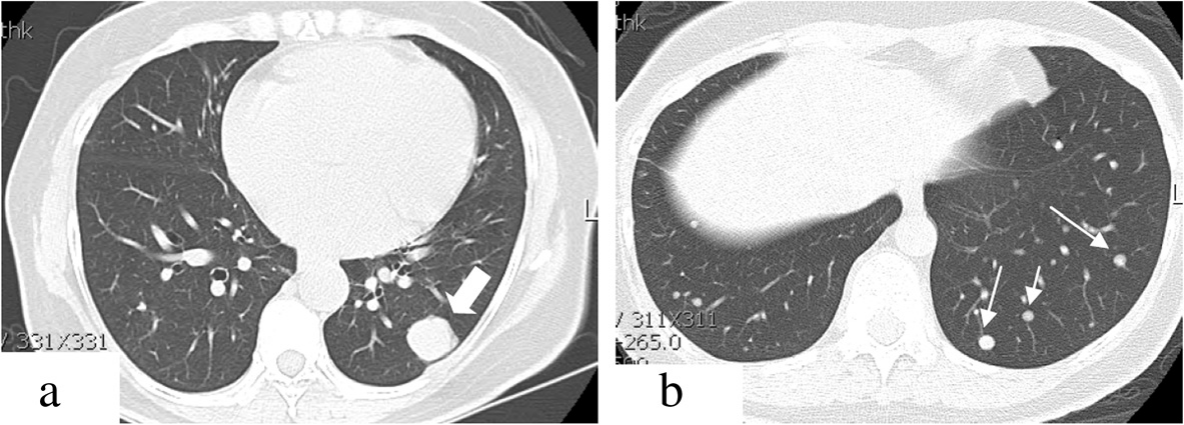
Chest computed tomography (CT) shows multiple pulmonary nodules. (a) Largest nodule measures 2.7 × 4.4 cm (thick white arrow). (b) Small variable nodules are seen in both lungs (narrow white arrow).
